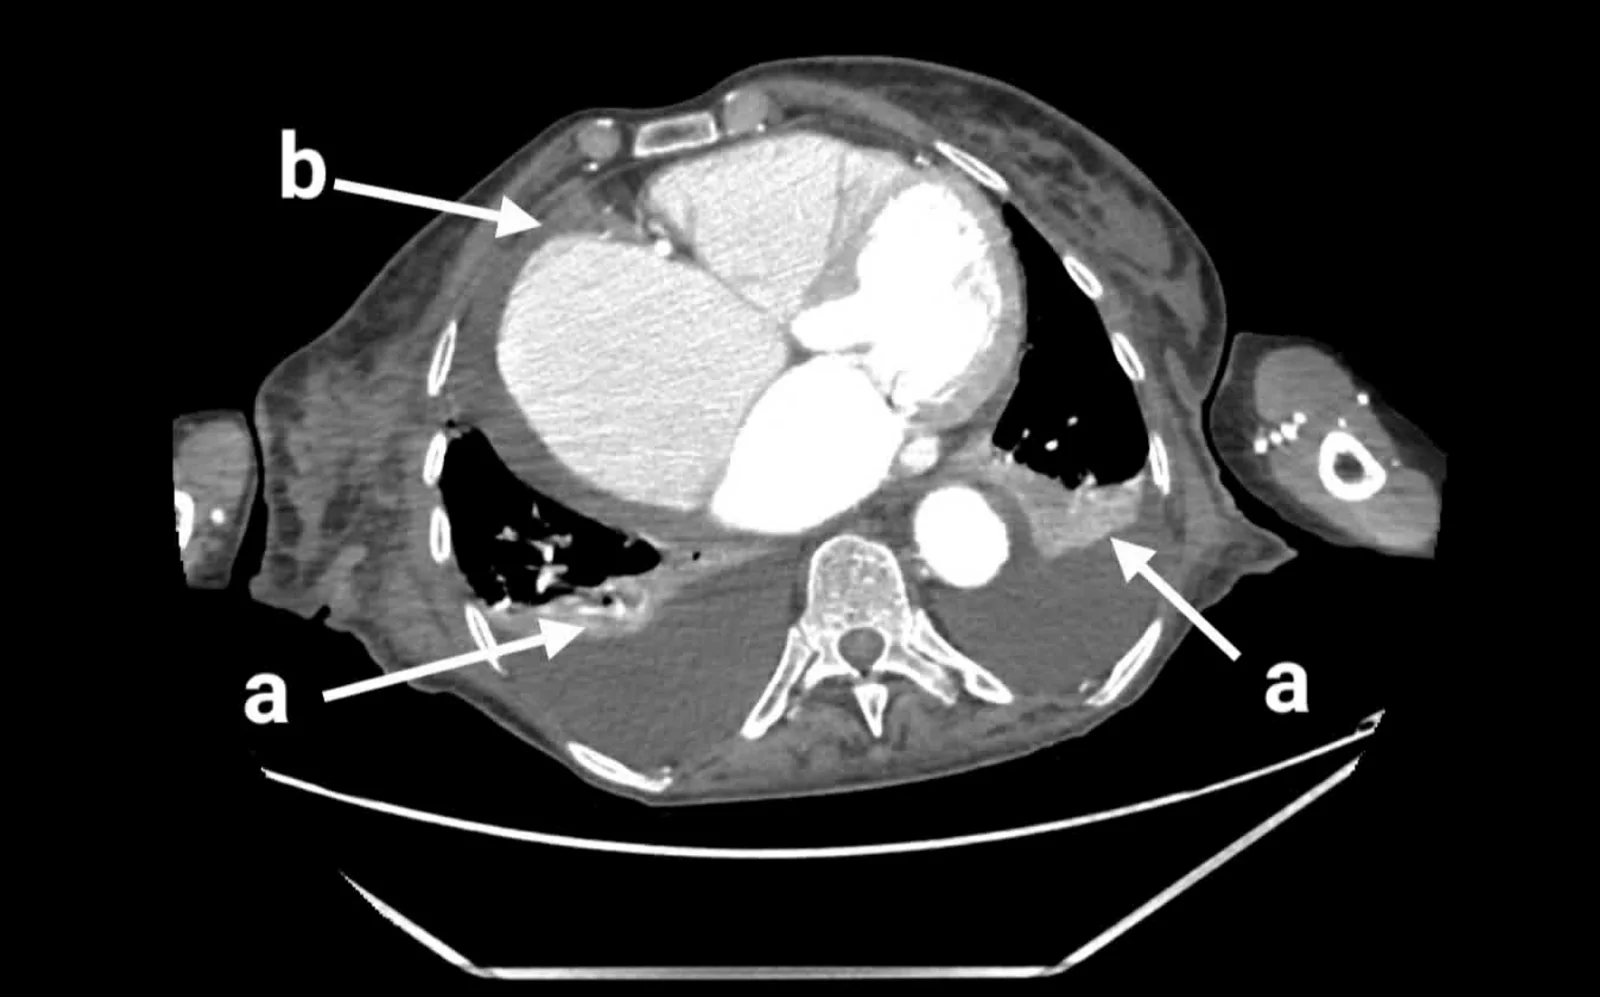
Contrast-enhanced chest computed tomography findings Axial contrast-enhanced chest computed tomography image obtained during admission showing bilateral pleural effusions with adjacent dependent atelectatic or consolidative change (arrows a), pericardial effusion along the anterior cardiac contour (arrow b), and marked enlargement of the cardiac chambers. These findings document the substantial cardiopulmonary fluid-overload context that provided a competing explanation for dyspnea; they are not diagnostic of CAD.
